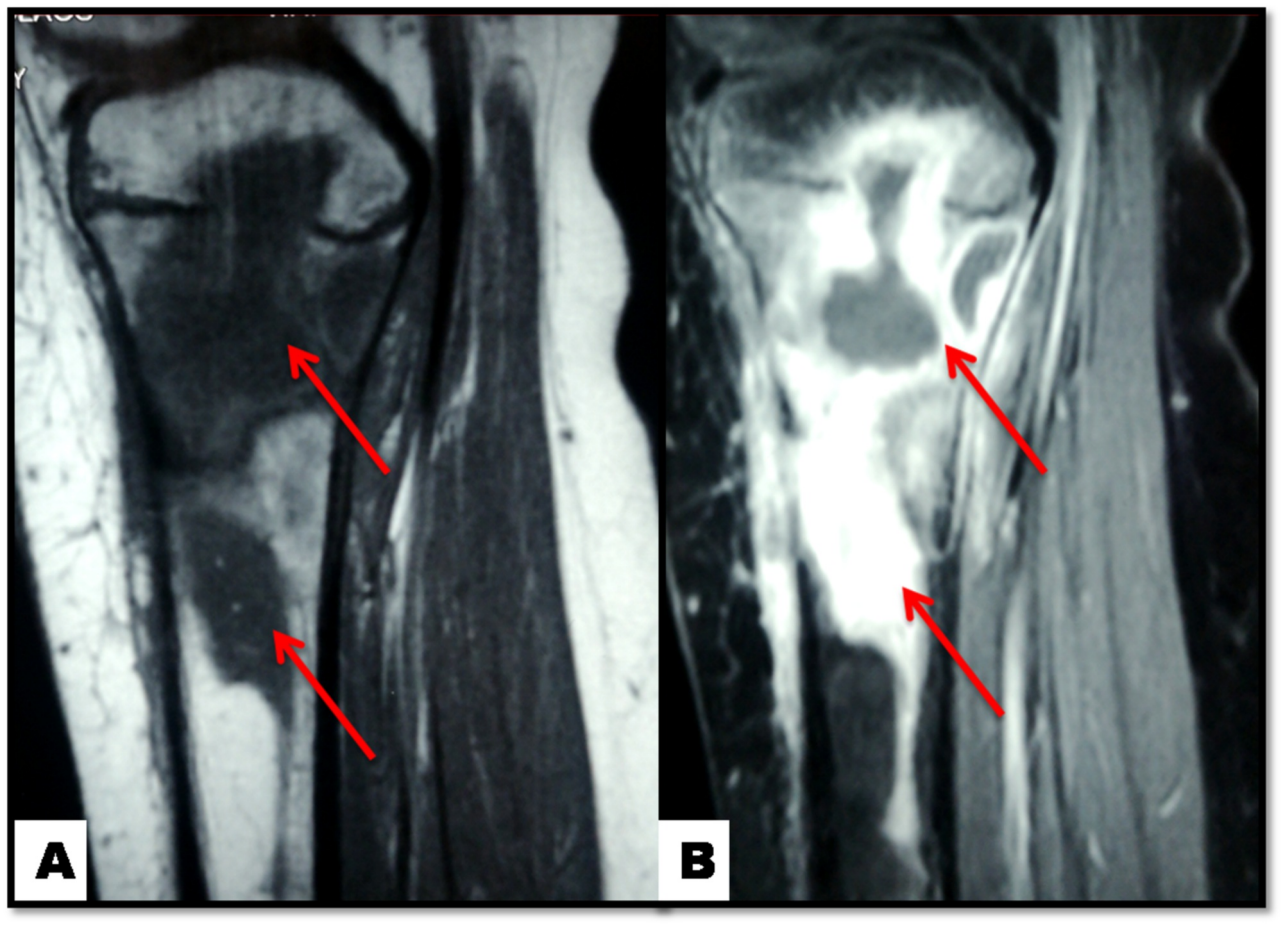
Knee MRI: osteomyelitis of the proximal tibia (red arrows) A: sagittal T1-weighted sequence; B: sagittal T2-weighted sequence
